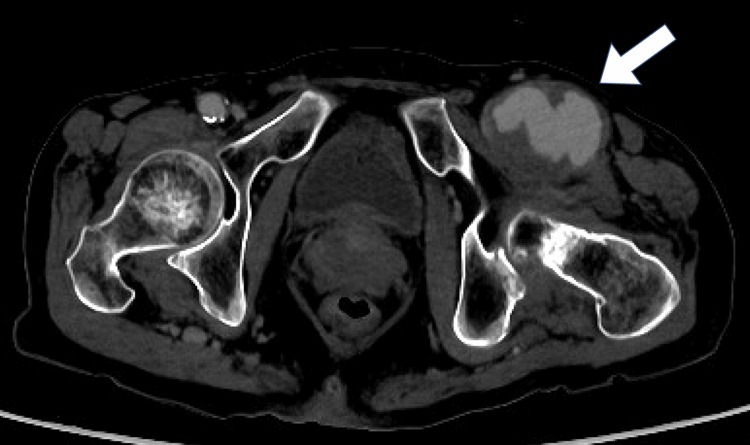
Enhanced CT at the time of emergency surgery reveals an enlarged pseudoaneurysm The allow indicates a pseudoaneurysm enlarged to 46 x 56 mm. CT: computed tomography
